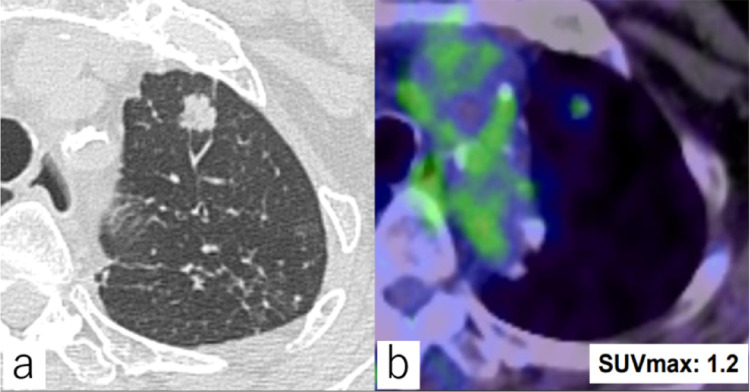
Representative case with radiological features suggesting low tumor aggressiveness. (a) A 65-year-old woman, diagnosed with adenocarcinoma, presenting with a 1.4-cm tumor in the left S1+2 tumor, with a consolidation-to-tumor (C/T) ratio of 0.71. (b) The tumor showed low FDG uptake (SUVmax, 1.2). She underwent a left superior segmentectomy and has remained recurrence-free for 37 months after surgery
